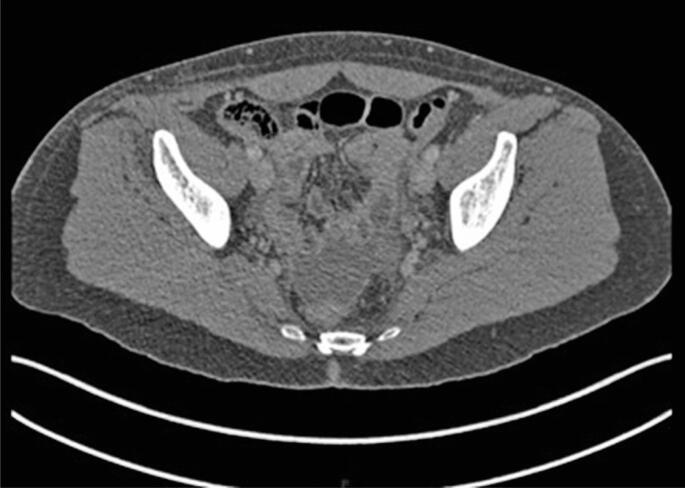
Abdominal computed tomography image showing free fluid in the pelvis. Image was generated during the investigation of possible urinary leakage
